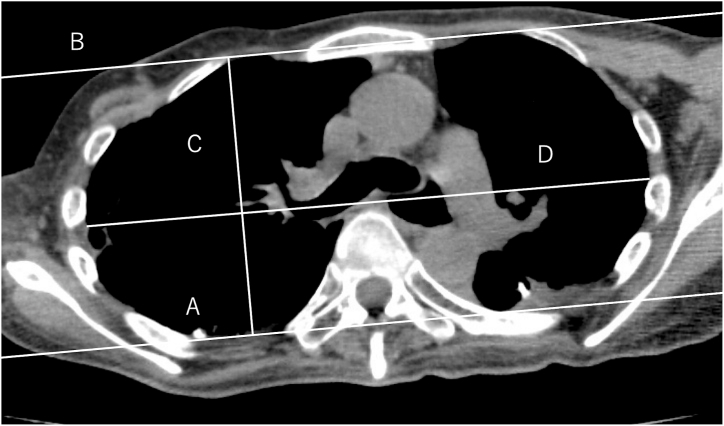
Measurement of thoracic flattening Axial chest CT at the sixth thoracic vertebra level showing marked flattening of the thoracic cage. The flattening index (anteroposterior diameter/transverse diameter) was 0.501, indicating severe platythorax. Lines A and B represent the posterior and anterior borders of the bony thorax, respectively. C indicates the anteroposterior diameter, and D indicates the maximum transverse diameter.
